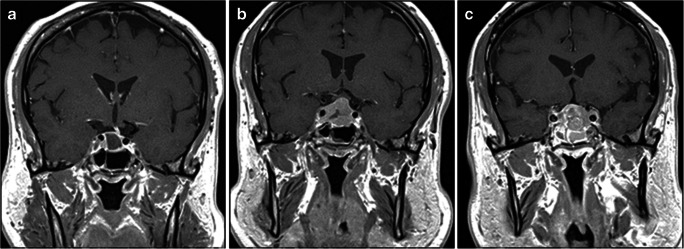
(Patient 1) Preoperative coronal post-contrast T1 MRI documents spontaneous resolution of the sellar lesion with a residual largely sellar cystic lesion causing deviation of the pituitary stalk to the left. There is minimal bulging into the suprasellar cistern but no compromise on the optic chiasm (A). Coronal post-contrast T1 imaging after 4 months shows interval deterioration in appearances with a recurrent solid enhancing sellar mass invading the right cavernous sinus. There is also worsening extension into the suprasellar cistern with displacement of the optic chiasm superiorly (B). Post-contrast coronal MRI after surgery and radiotherapy shows a recurrent solid enhancing sellar mass invading the cavernous sinuses bilaterally and with suprasellar extension (C)
